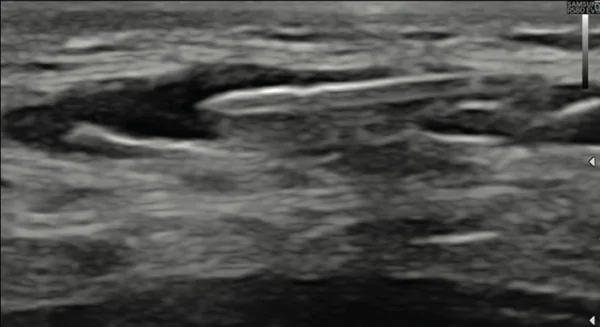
Longitudinal ultrasound image showing access of the inguinal lymph node with a 23G needle.
